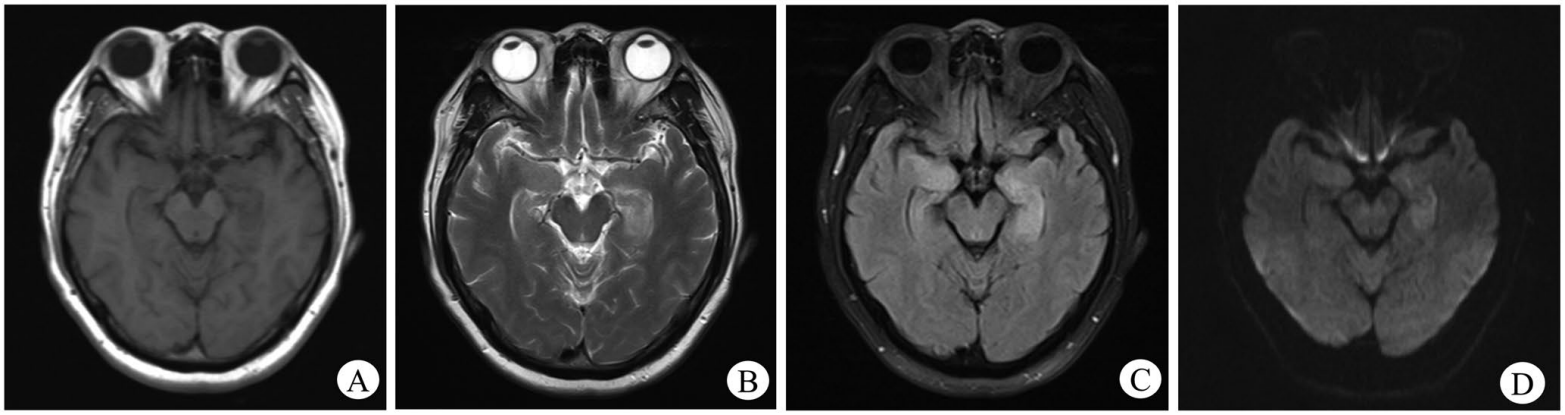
Bilateral hippocampal abnormalities on MRI in LGI1‐AE. Irregular patchy abnormal signals are seen bilaterally in the hippocampal region of the deep temporal lobes, with slightly low signals in T1WI (A) and slightly high signals in T2WI (B), T2WI‐FLAIR (C) and DWI (D).
